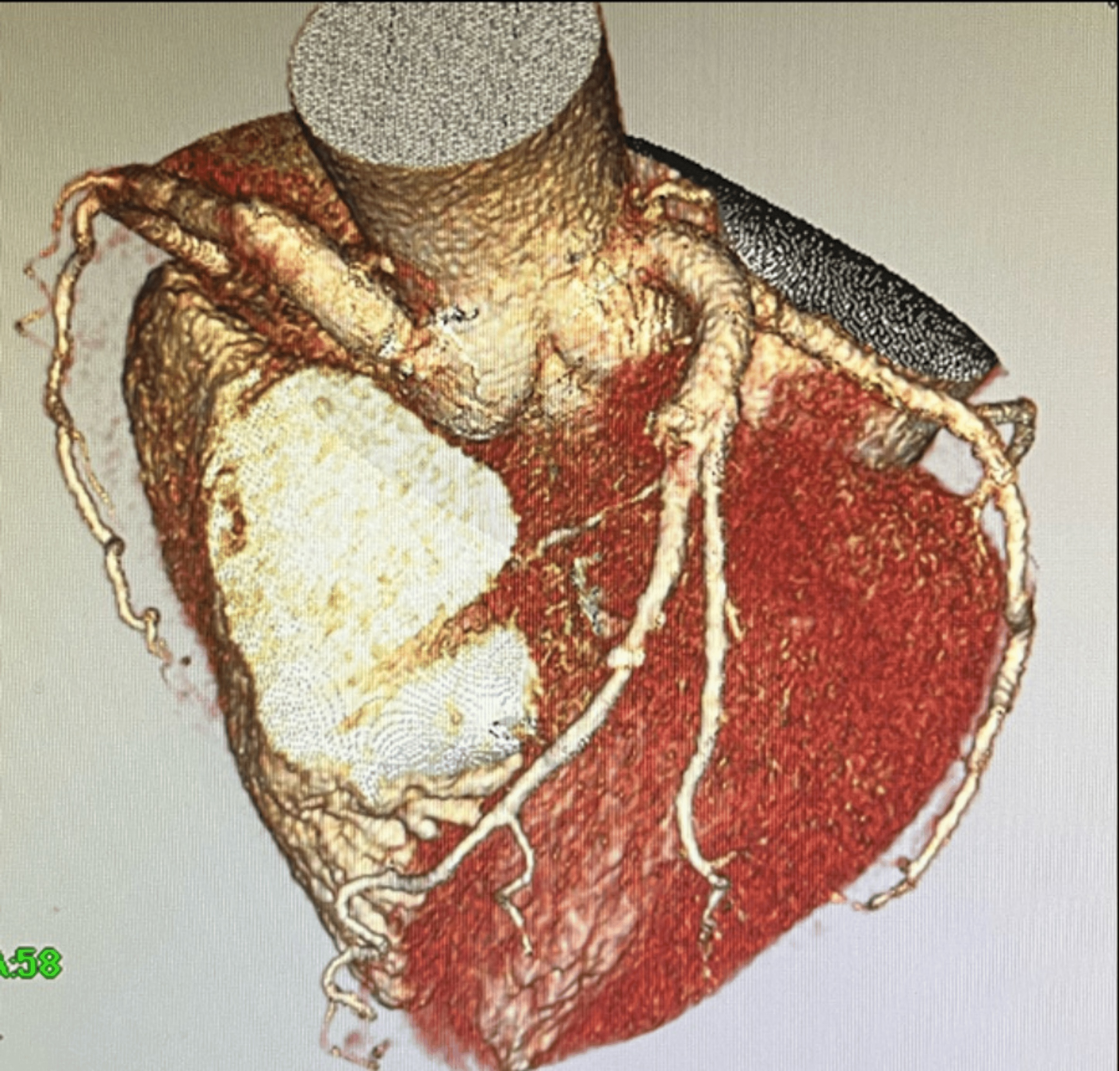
Postoperative coronary 3D CT image Postoperative coronary CT revealed the disappearance of the coronary artery aneurysms, abnormal vessels, and the coronary artery-to-pulmonary artery fistula. CT, computed tomography
